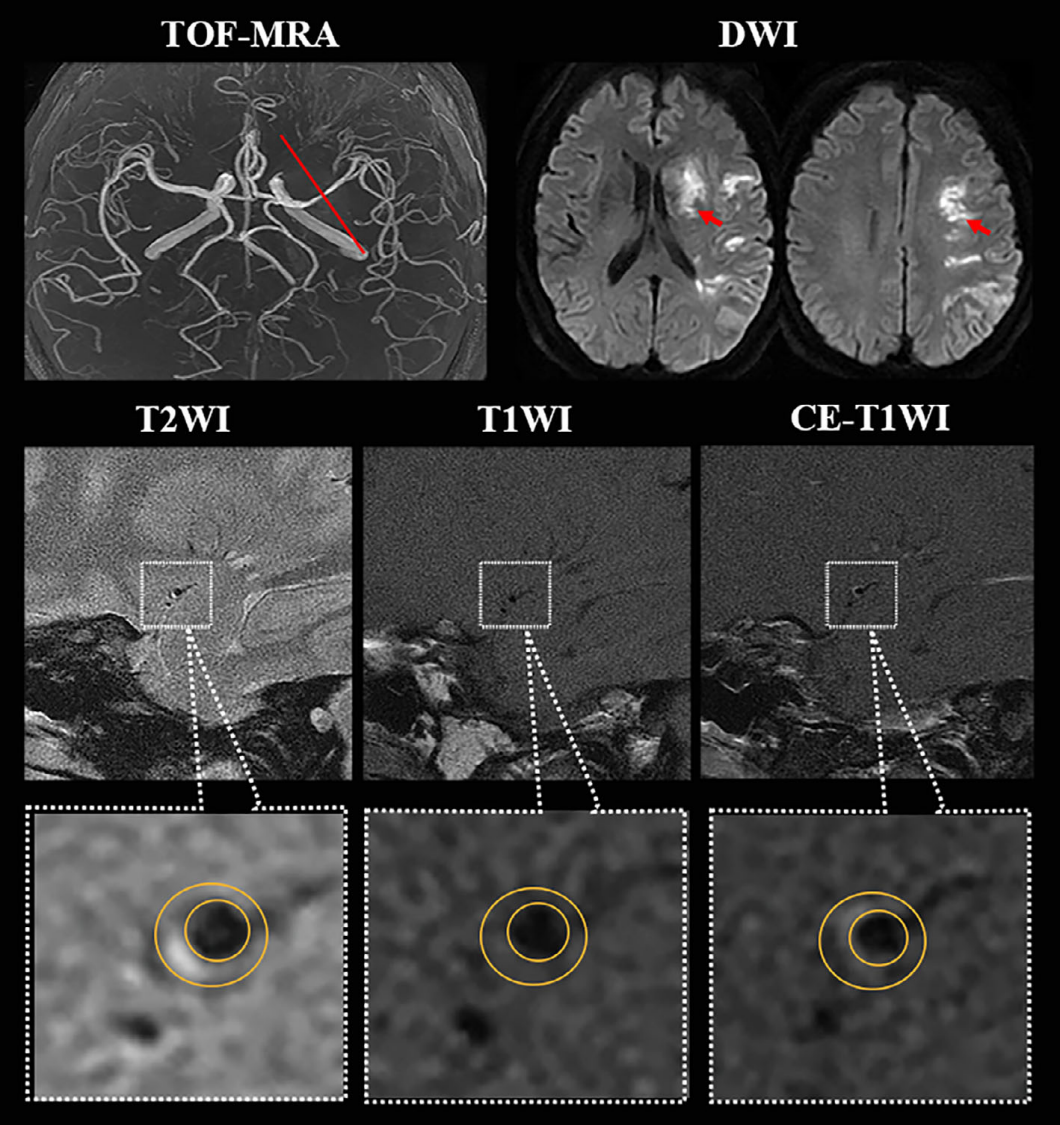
Higher resolution magnetic resonance imaging (hrMRI) images showing intracranial atherosclerotic plaque on middle cerebral artery. A 53‐year‐old man with hypertension for 10 years and without previous blood pressure management having acute ischemic stroke on the left cerebrum; TOF‐MRA demonstrated a mild stenosis (<50%) located on M1 segment of left MCA, and DWI showed a sheet acute infarcts; hrMRI images including T2WI, T1WI, and CE‐T1WI at the most stenotic site visualized the outer wall boundary (large yellow circle) and the lumen (small yellow circle), and the hrMRI parameters (minimal luminal area = 2.94 mm^2^; plaque volume = 18.98 mm^3^; plaque burden = 76.3%; remodeling ratio = 67.1%; eccentricity index = 0.51; enhancement ratio = −5.3%) were obtained; after hospitalizing management under high‐risk factor control, he had a favorable outcome with 90 mRS being 1.
